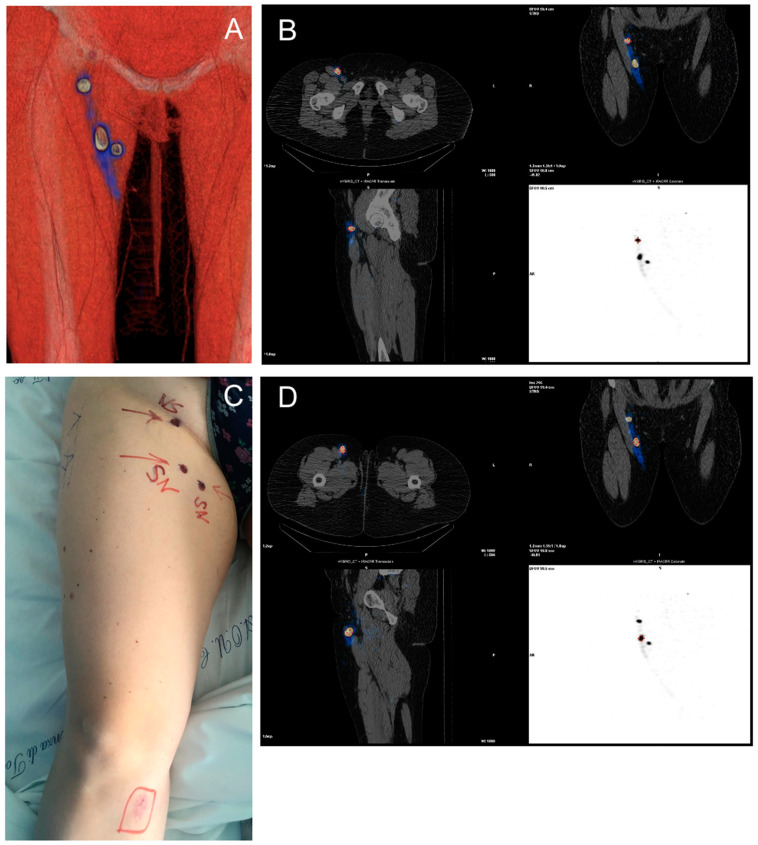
(Case 2) (A) A 3D image of the inguinal sentinel lymph node. (B–D) SPECT/CT rendering with a view of lymph nodes in CT reconstruction. (C) Preoperative image with skin marking of lymph nodes.
